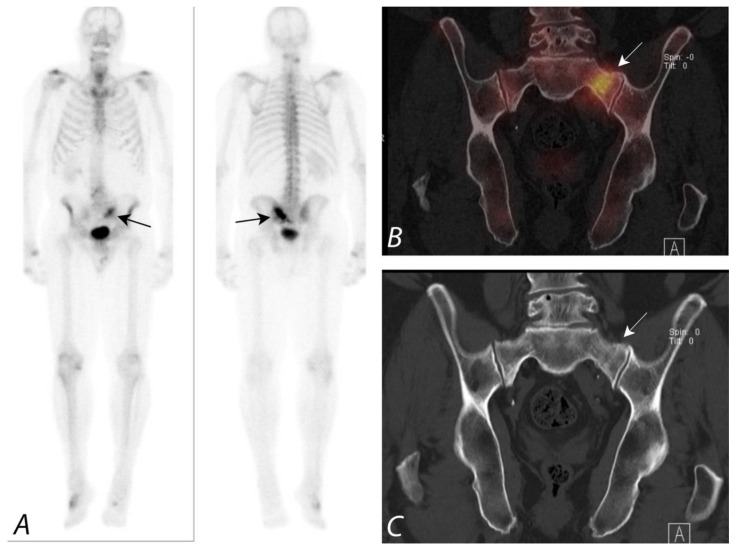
Anterior and posterior whole body ^99m^Tc MDP bone scan planar images (A) from a Tc-99m MDP bone scan in a 68-year-old male with prostate cancer and new pelvic pain demonstrate focal uptake (black arrows) localizing to the left sacral region. Coronal CT and fused SPECT/CT images (B,C) demonstrate the uptake localizing to a vertical insufficiency fracture of the left sacral ala, which demonstrates osteoblastic activity, confirming a healing insufficiency fracture and not metastatic disease.
